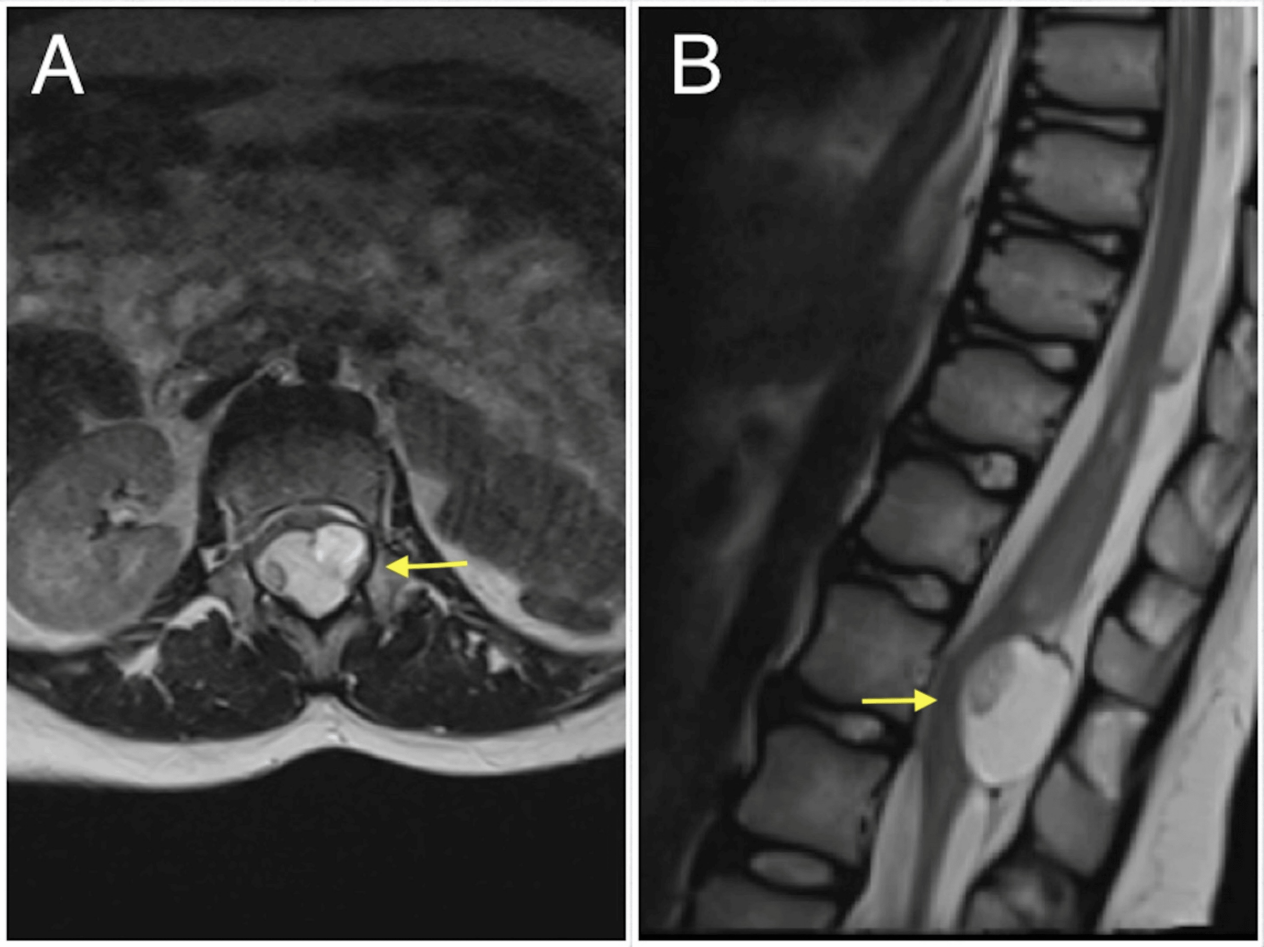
(A) Axial and (B) sagittal T2-weighted MRI scans of the spine showing a hyperintense cystic lesion at the level of the conus medullaris (yellow arrow) with a small nodular isointense area inside.
